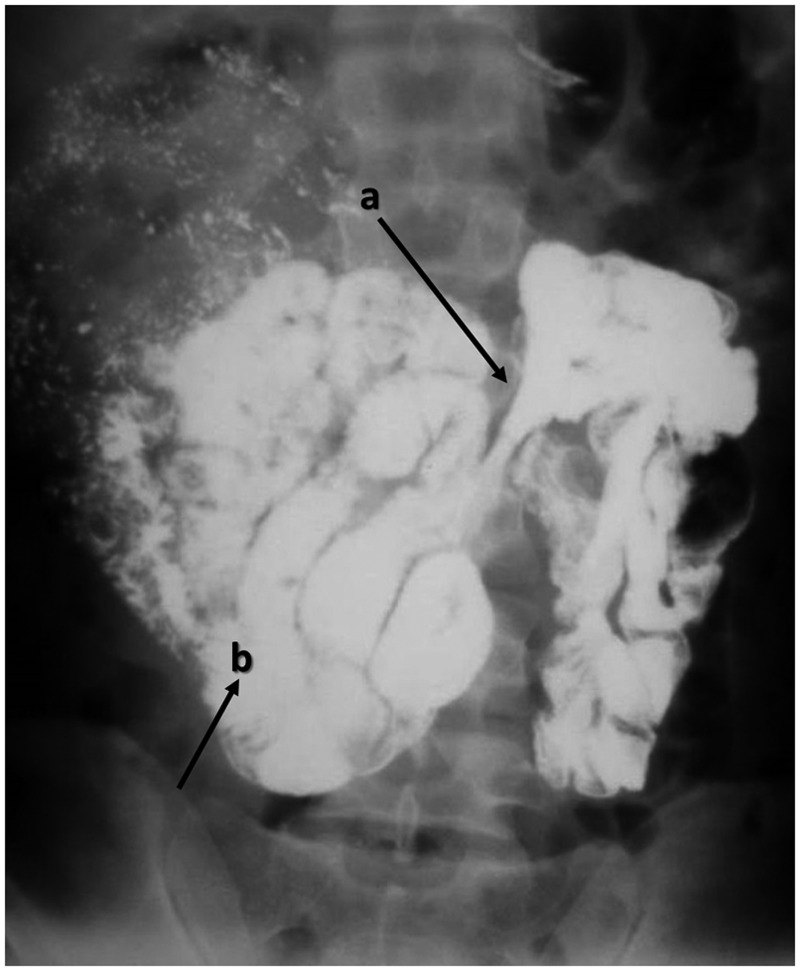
A plain erect abdominal X-ray of Case 2, showing right paraduodenal hernia. The opening of the fossae of Waldeyer is marked by arrow a). small bowel loops are clustered to the right of the midline (marked by arrow b), in a well circumscribed hernial sac.
